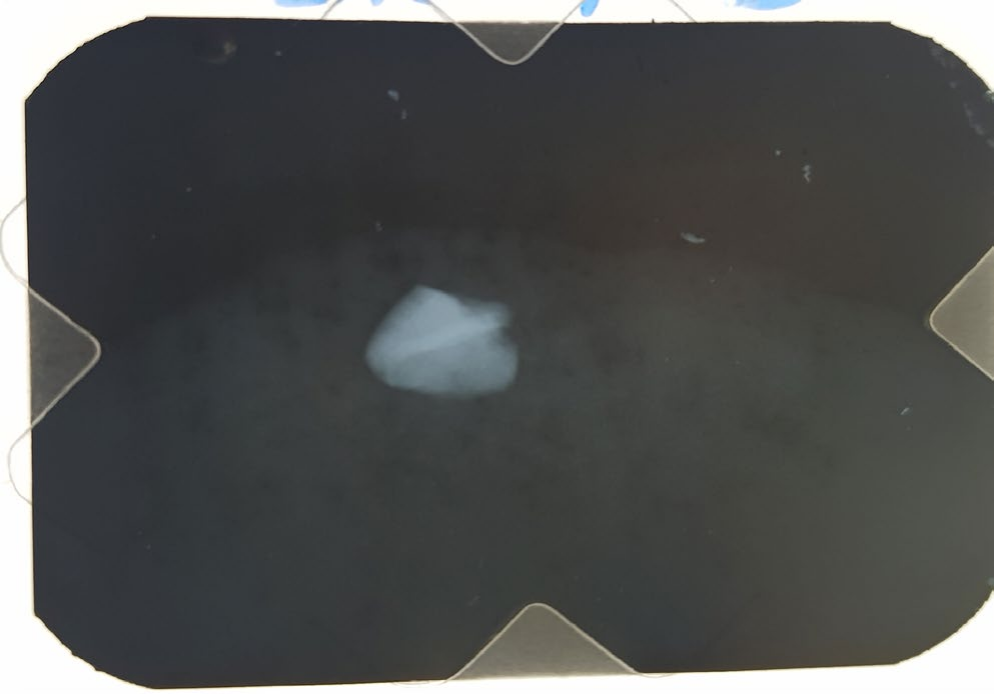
Extraoral radiograph of lower lip
